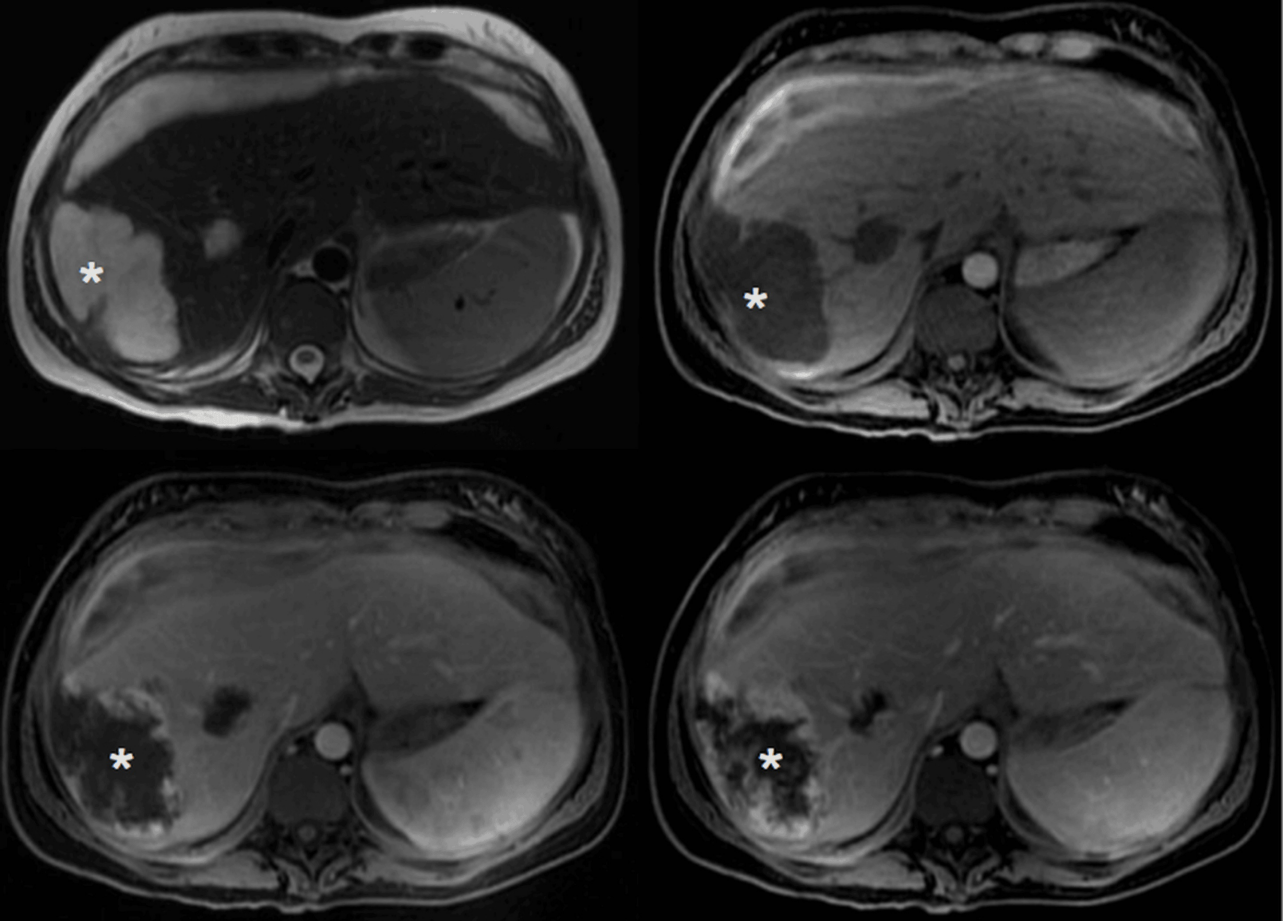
Magnetic resonance imaging shows hepatic subcapsular hematoma and hemangiomas in the right hepatic lobe
